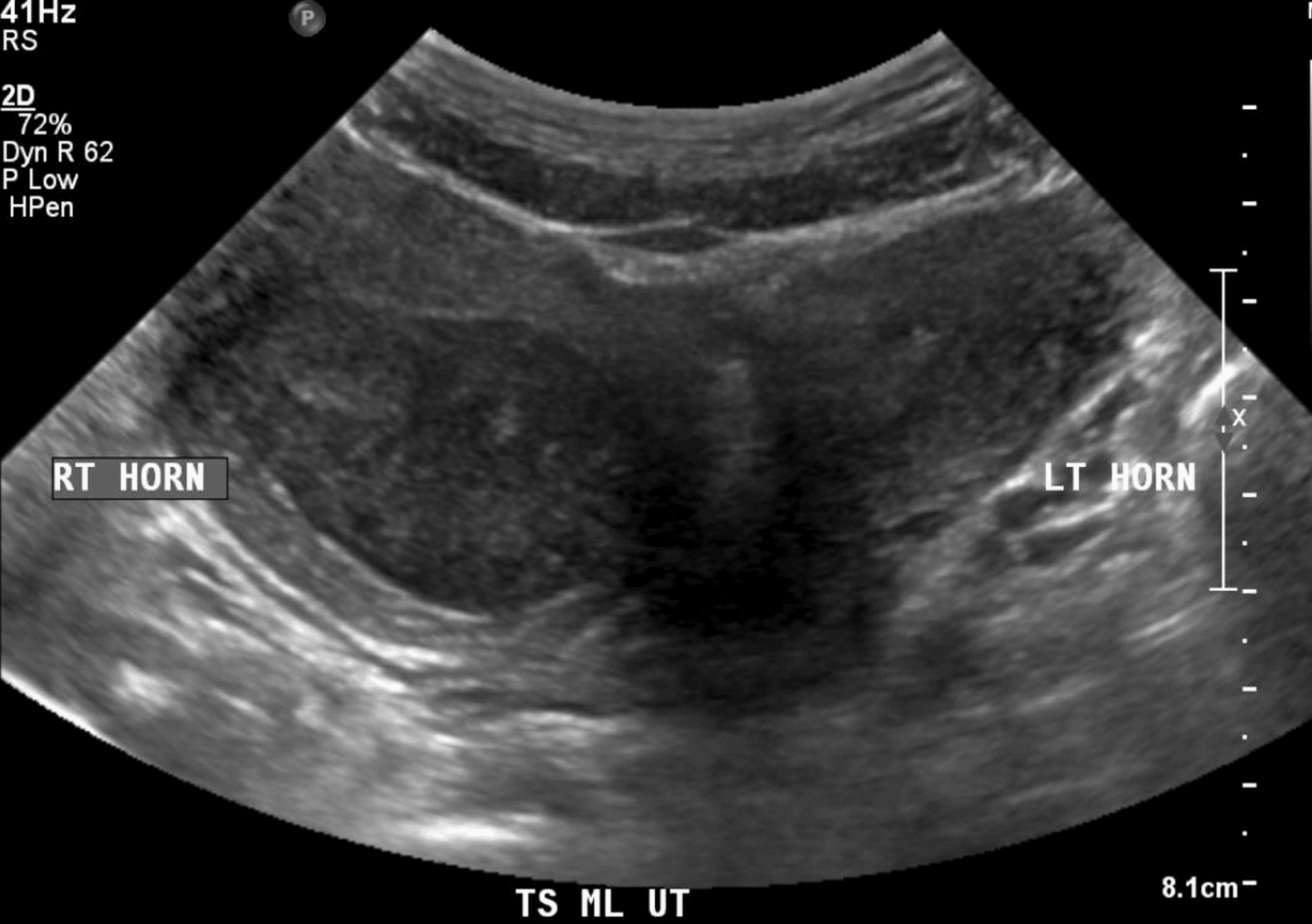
Transverse view of the uterus on transabdominal ultrasound showing uterine didelphys
